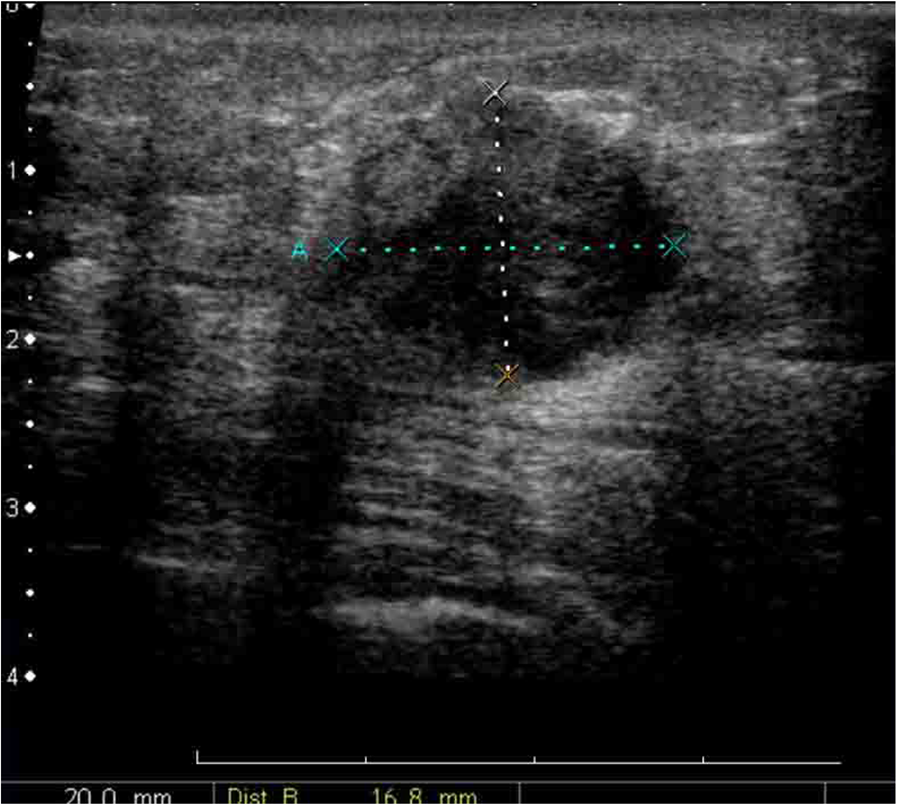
Ultrasonography shows an inhomogeneous, hypoechoic nodule measuring 20mm×17mm.
